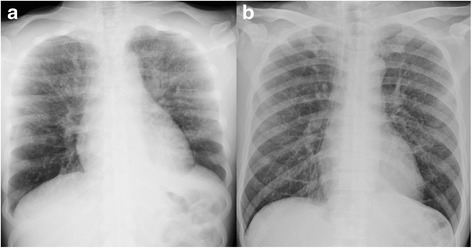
Chest X-ray. Chest X-ray at admission showing consolidation in the upper lungs (a); the abnormal shadow disappeared 4 weeks after admission (b)
